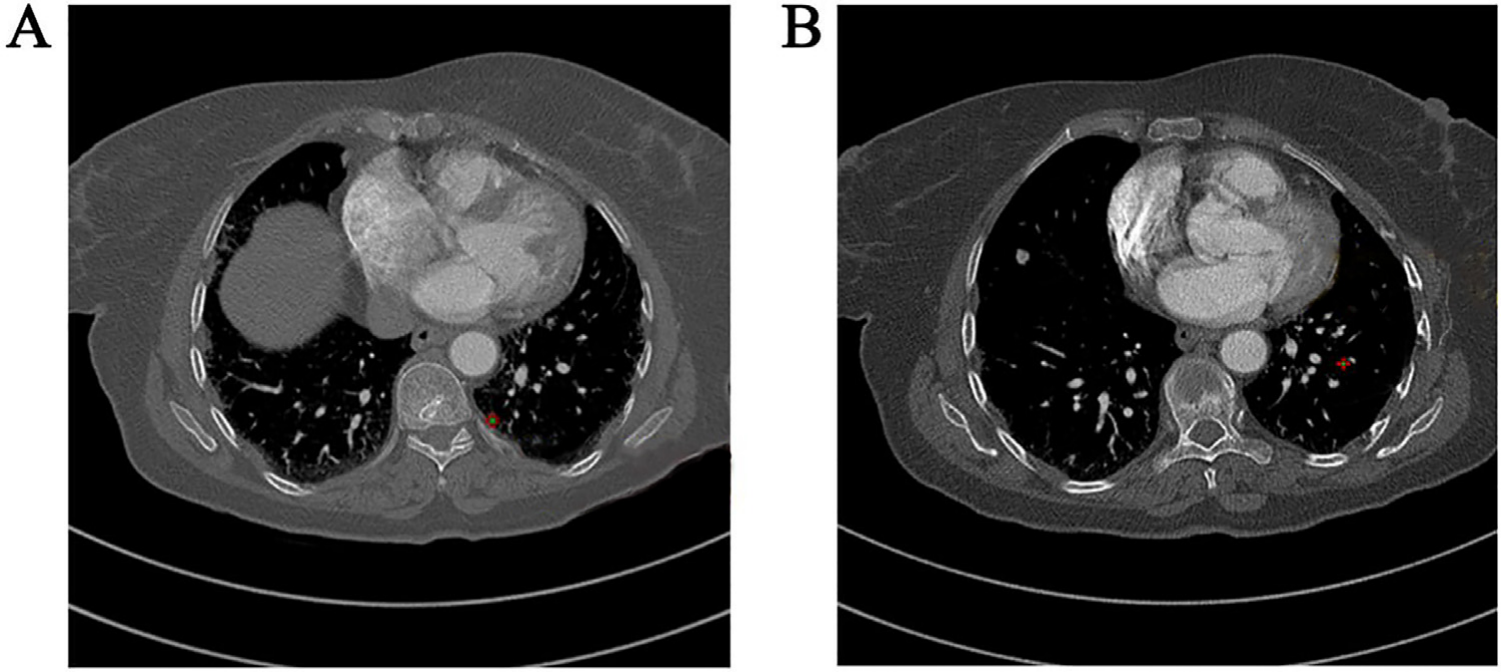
Chest CT scan showing (A) small nodule in the left lower lobe with faint calcification, measuring 476 HU in density, (B) Small nodule in the left lower lobe with frank calcification, measuring 880 HU in density.
